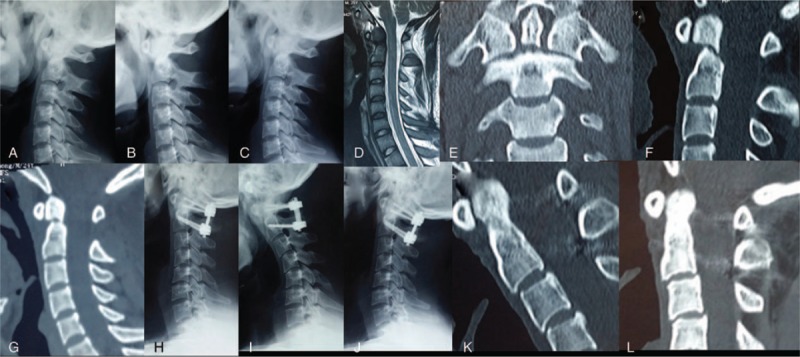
Male, 29 years old, duration from injury to operation was 8 days. (A–C) Preoperative lateral, flexion, and extension X-ray showed odontoid fracture. (D) Preoperative MRI showed odontoid fracture without spinal cord compression. (E, F) Preoperative CT showed type II B odontoid fracture, with fracture anterior displacement = 8.1 mm. (G) Reduction of the displaced odontoid fracture was attempted using the Mayfield head-holding device. (H–J) Postoperative lateral, flexion, and extension X-ray showed posterior C1 lateral screw-C2 pedicle screws fixation. (K) Three-month follow-up CT scan showed bridging bone across the odontoid fracture site in sagittal CT-scans in neutral head position. (I) Twelve-month follow-up CT scan showed evident bridging bone across the odontoid fracture site in sagittal CT-scans in neutral head position.
